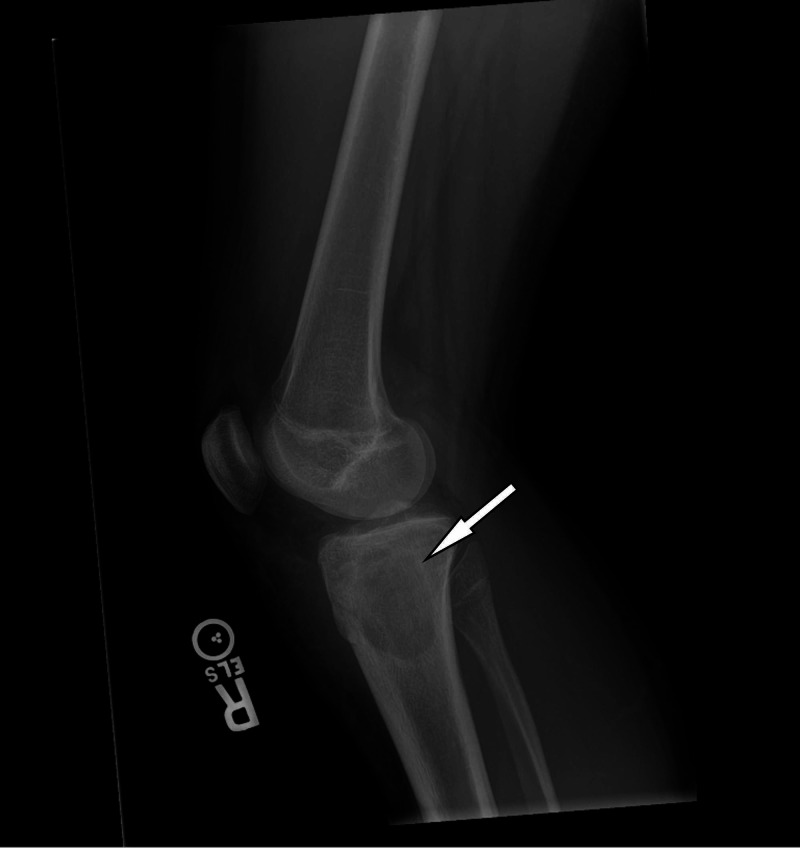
Lateral radiograph, with arrow demonstrating a lucency in the proximal right tibia.
